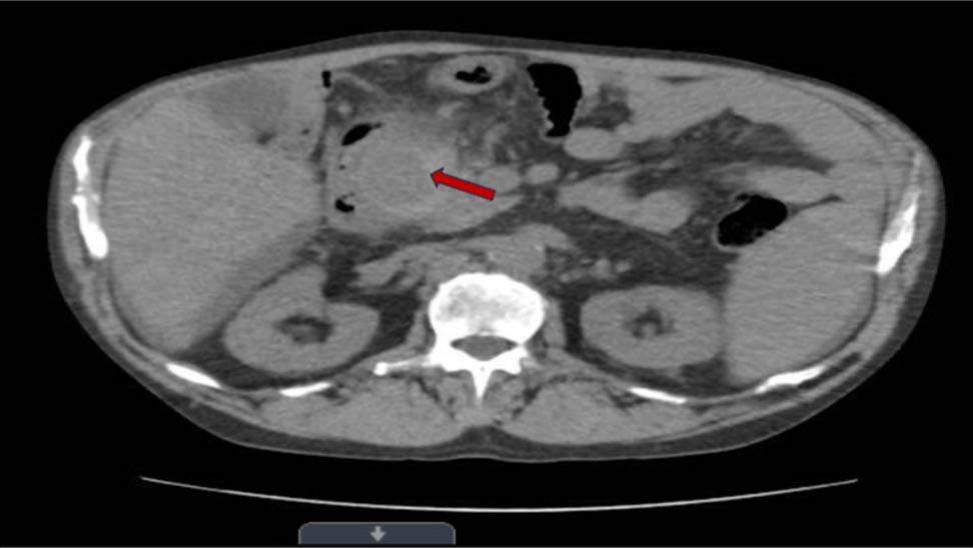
Axial computed tomography abdomen without intravenous contrast demonstrating peripancreatic inflammation around the pancreatic head. A presumed pancreatic pseudocyst is indicated (red arrow).
